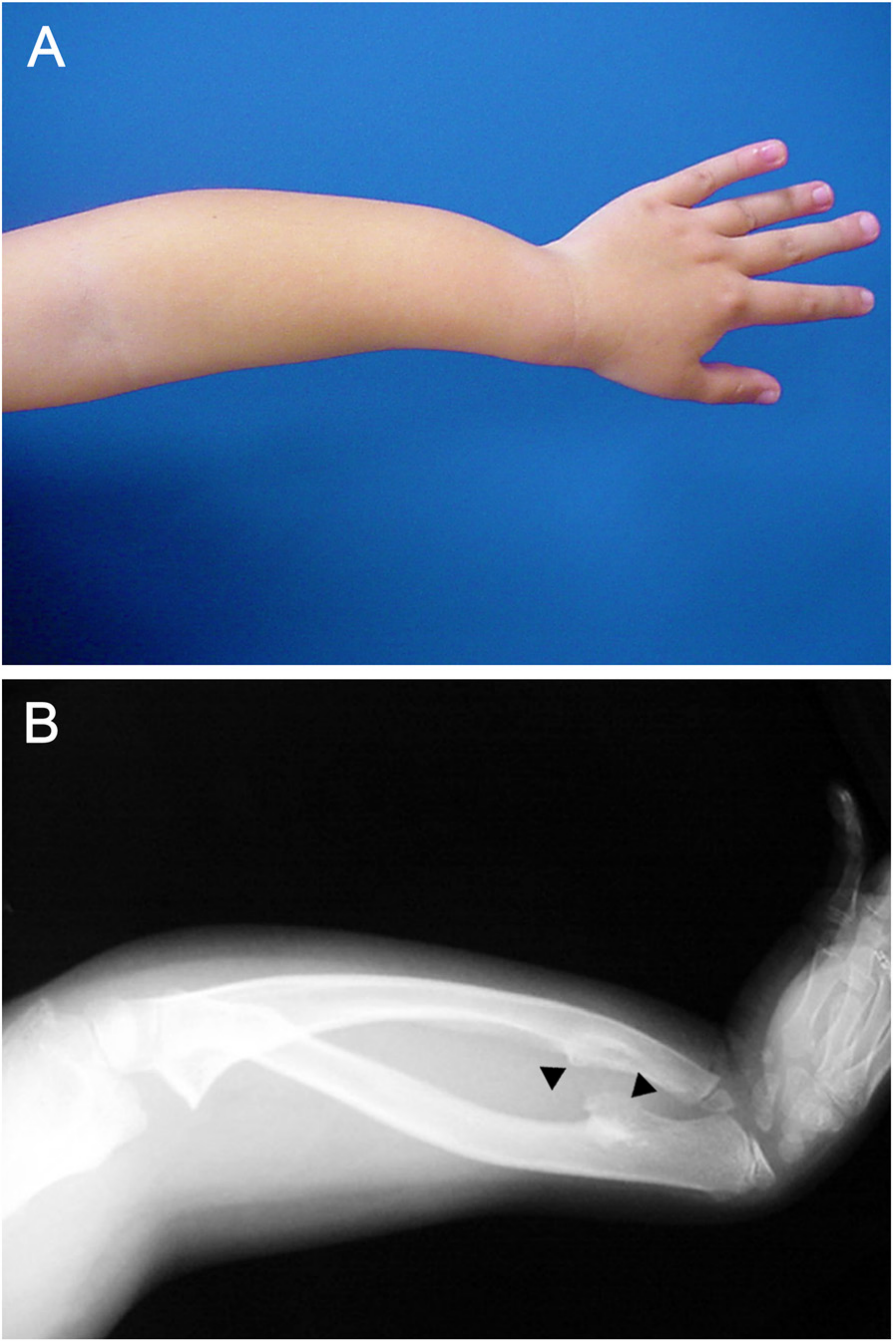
Manifestations of multiple osteochondromas (MO) in a patient. A, Photograph of a 7-year-old patient with MO demonstrates marked forearm deformities. B, In his radiograph, the most common type of deformity is seen: a combination of relative shortening of the ulna, bowing of the radius and ulna, increased ulnar tilt of the distal radial epiphysis, and ulnar deviation of the hand (Courtesy of Mikel San Julian, MD, Department of Orthopaedic Surgery and Traumatology, University Clinic of Navarra, Pamplona, Spain).
